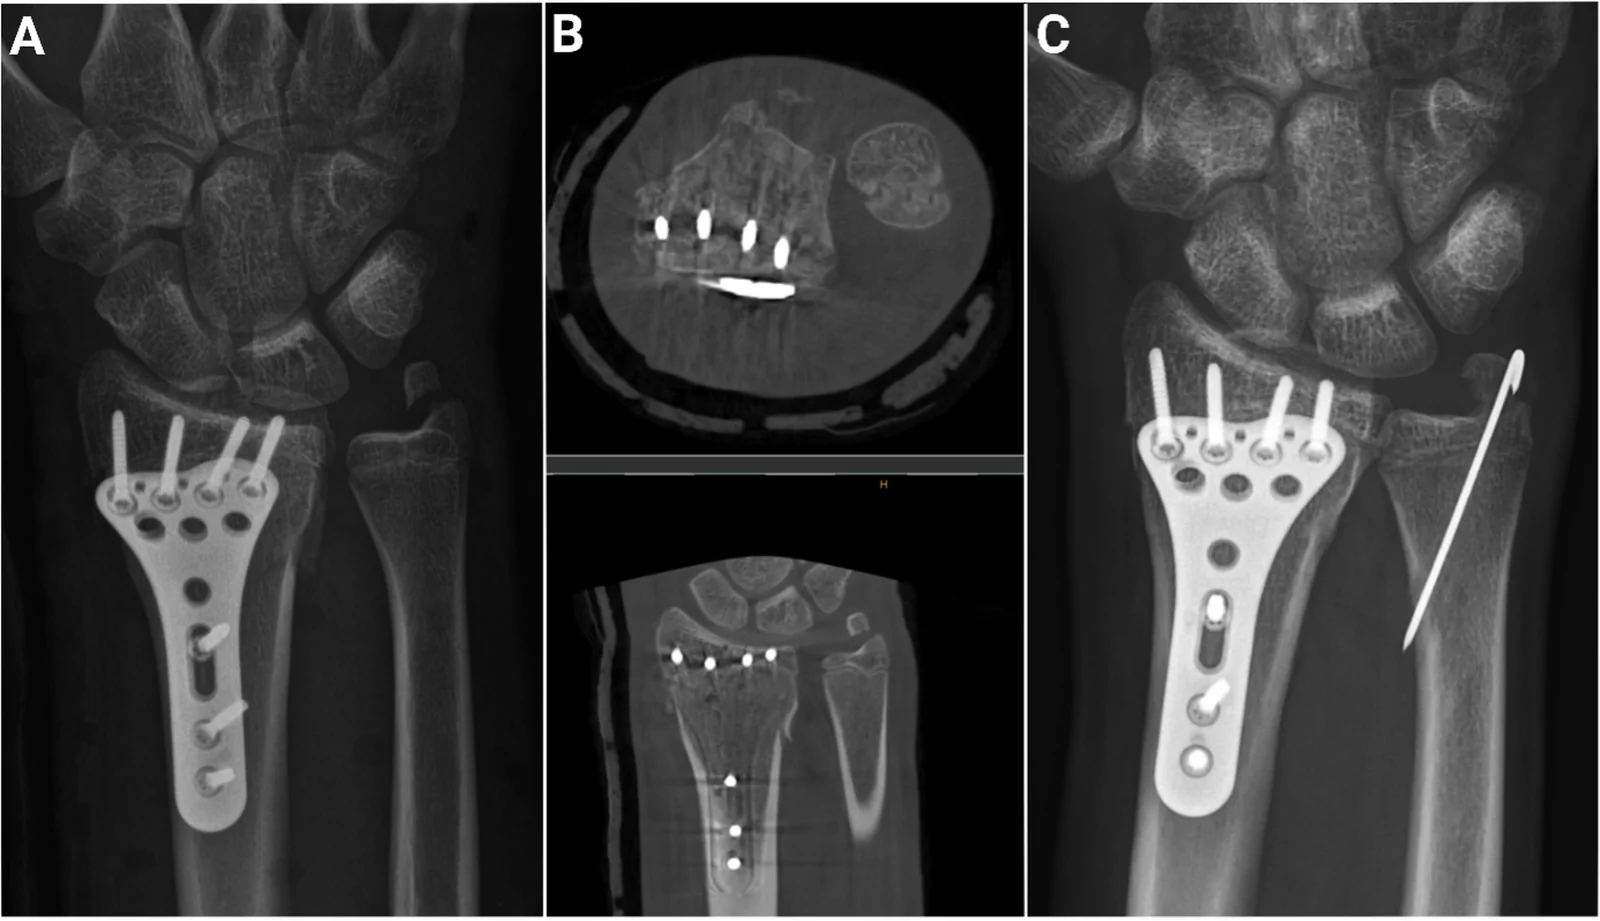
Demonstration of a clinical case. The distal radius fracture was stabilized using plate osteosynthesis (A). Postoperative computed tomography (CT) demonstrated persistent instability of the DRUJ (B). Restoration of distal radioulnar joint stability and radiological signs of early consolidation at 6 weeks postoperatively were achieved by single K-wire osteosynthesis of the ulnar styloid (Fig. 1C)
